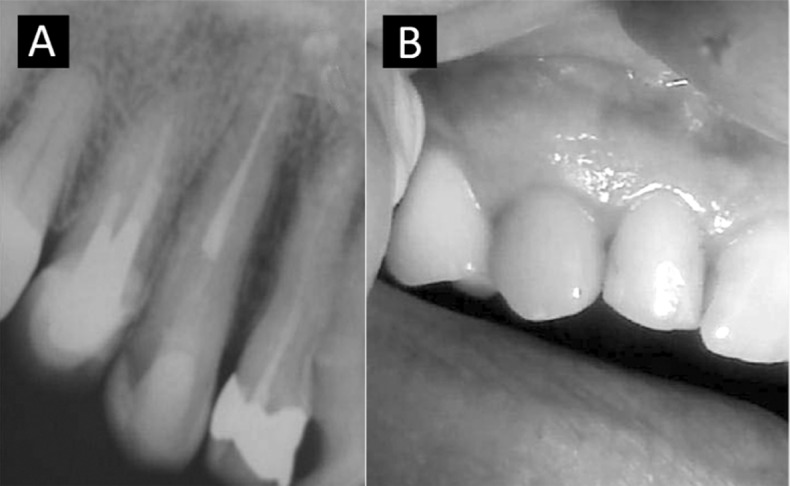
Two years follow-up; A) Radiographic; B) Clinical
